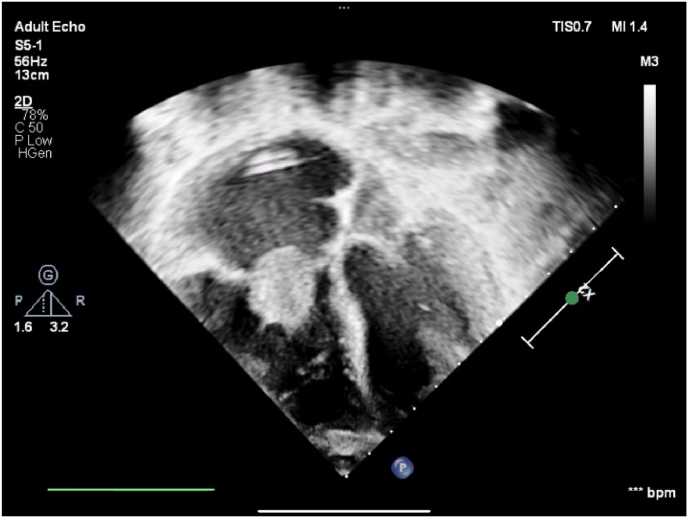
Echocardiogram showing normal cardiac anatomy and function. A 2.5 × 1 cm thrombus is seen in the right ventricle. Tip of a left subclavian catheter is visible in the right atrium.
